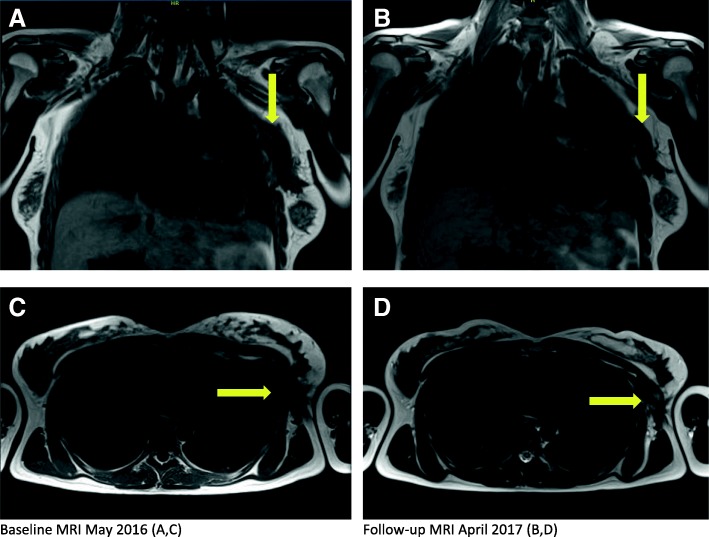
Radiological evaluation. Magnetic resonance imaging (t1 weighted) demonstrating frontal (a, b) (yellow arrows indicating cranial tumour border) and axial (c, d) (yellow arrows indicating medial tumour border) sections of pretreatment (a, c) and follow-up (b, d) examination 19 months after completion of intratumoural LTX-315 injections
